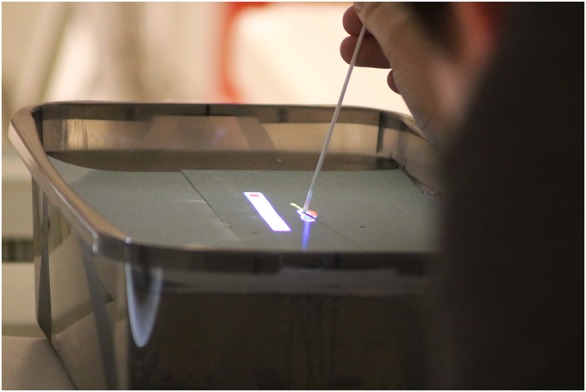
Exemplary view over the user's shoulder of the AR needle navigation inside the MRI bore. A combination of both 2D and 3D concepts is shown
